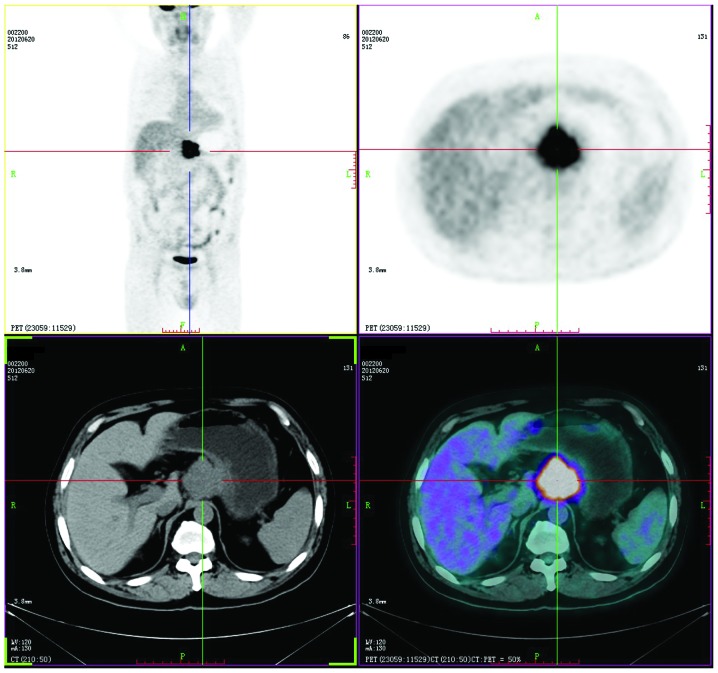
Positron emission tomography-computed tomography demonstrated a hypermetabolic lesion (standardized uptake value, 12.8) in the interspace between the liver and stomach. There was no additional fludeoxyglucose (18F) uptake, which indicated a primary site in other organs.
